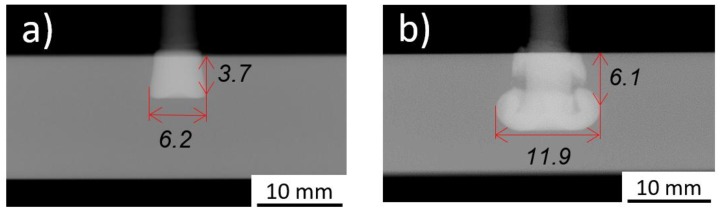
X-ray tomography of: (a) Condition 1 with lowest energy input, E_M_ = 24 J (RS = 18,000 rpm; FT = 1.6 s; FoT = 1 s; FF = 2000 N; FoF = 5100 N); and (b), Condition 16 with highest energy input, E_M_ = 208 J (RS = 20,000 rpm; FT = 2 s; FoT = 2 s; FF = 3000 N; FoF = 5100 N).
